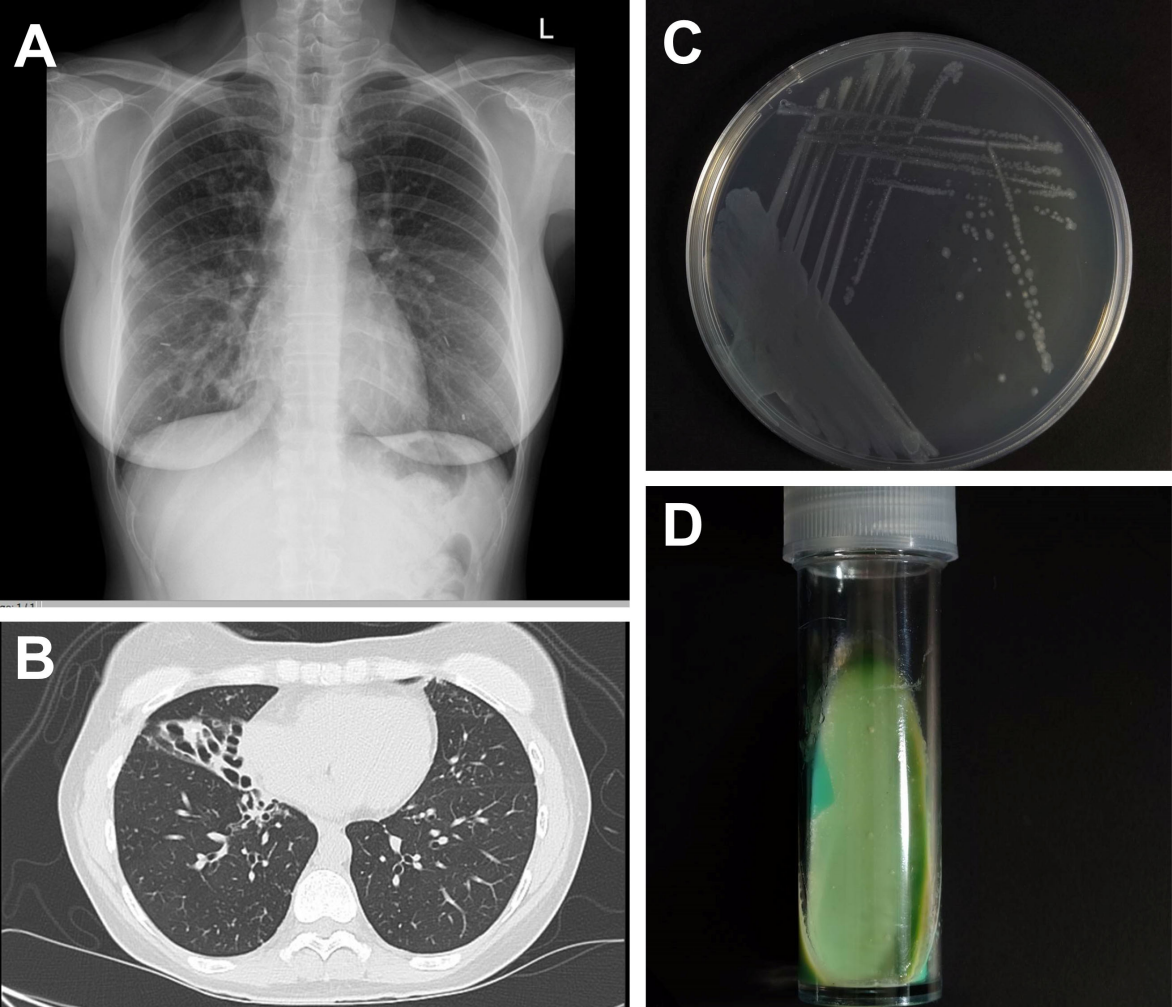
Investigations of *Mycobacterium avium* complex. (A) Chest radiography shows interstitial infiltration at right middle lung field and (B) computed tomography demonstrates centrilobular with tree-in-bud appearance and multiple pulmonary nodules along with cylindrical bronchiectasis. The growing colonies of *M. avium* subspecies avium ATCC 25921 on (C) Middlebrook 7H10 medium and (D) Löwenstein–Jensen medium that grew at 35 C for 3 weeks.
